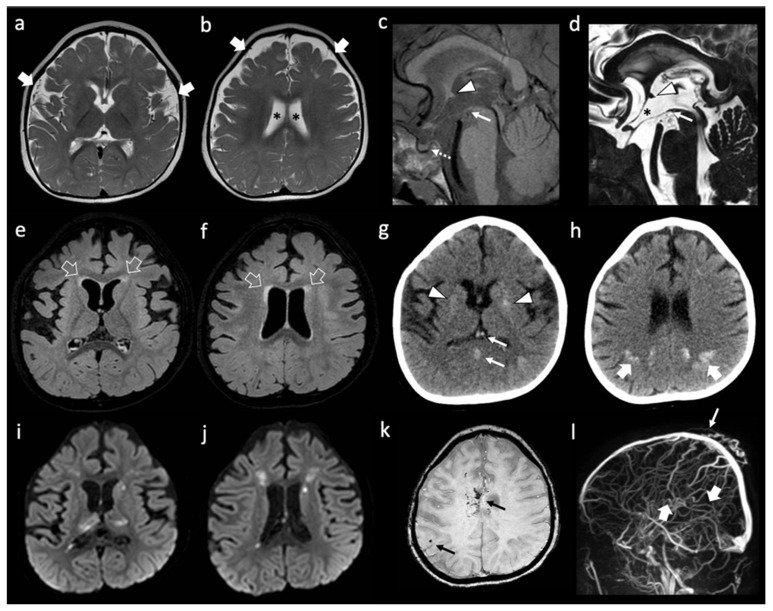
Neuroimaging features of patient A. (a,b) Brain MRI with axial T2-weighted images performed at 1 year of life showing enlargement of the frontal and temporal subarachnoid spaces (thick white arrows) and of the lateral ventricles (black asterisks). Note, the simplified gyration of the cortex, especially in the fronto-temporal lobes. (c–f) brain MRI performed at 3 years of age with sagittal T1-weighted (c) sagittal DRIVE (d) and axial FLAIR (e,f) images reveal dysmorphic anterior commissure (white arrowheads), thin anterior recesses of the third ventricle (black asterisk), absent/dysmorphic mamillary bodies (white arrows), and absent posterior pituitary bright spot (dotted white arrow). There is mild FLAIR hyperintensity of the frontal periventricular white matter (empty white arrows). (g,h) non-enhanced head CT images performed a few hours after the onset of right hemiparesis, four days after the previous brain MRI, reveal spontaneous hyper-densities of the internal cerebral veins and straight sinus (thin white arrows). In addition, there are subtle, widespread calcifications of the basal ganglia (white arrowheads) and well-demarcated, band-like calcifications of the subcortical parietal white matter (thick white arrows). (i–l) A brain MRI performed the same day of the CT, including diffusion-weighted images (i,j) susceptibility weighted imaging (k) and phase contrast MR venography (l) confirm the absence of blood flow in the straight sinus and internal cerebral veins (thick white arrows) with associated multiple venous ischemic lesions in the thalami and periventricular white matter. In addition, small bleedings are observed in the fronto-mesial and right frontal subcortical white matter (thin black arrows). Note, the parietal median sinus pericranii (thin white arrow).
